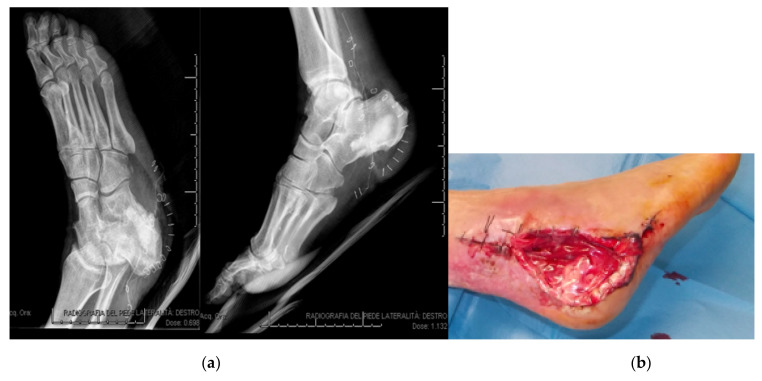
Postoperative X-rays (a) and photograph (b) after further skin and soft tissue debridement plus resection of the infected and necrotic bone and filling of the calcaneal bone void with absorbable local antibiotic carrier.
